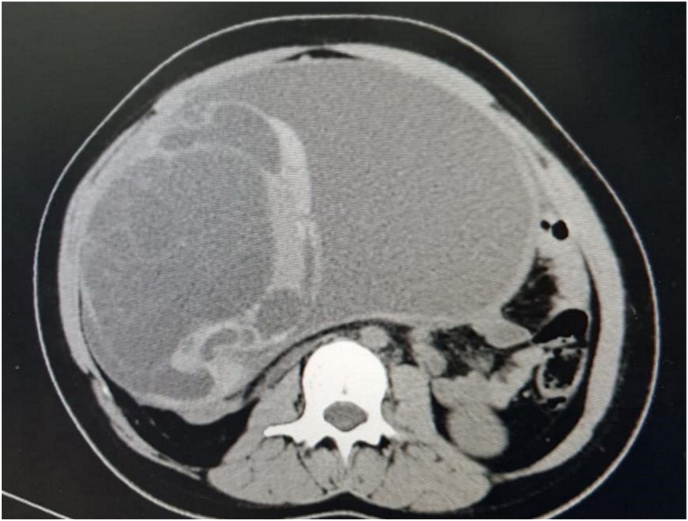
Selected image in the axial section of a contrast CT of the abdomen demonstrating a large complex non-fat containing cystic mass with multiple smaller locules of cysts with enhancing septations within, suggesting a multilocular cystic mass. There is no calcification within.
